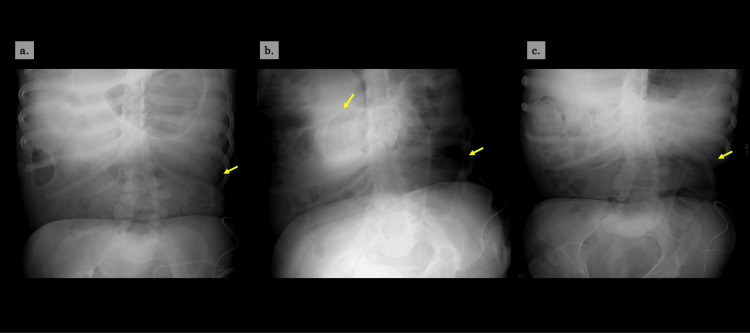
Changes in abdominal X-ray findings a. Postoperative day 7, no bowel dilatation b. Postoperative day 11 (before initiation of the stoma procedure), bowel dilatation extending c. Postoperative day 13 (after initiation of the stoma procedure), improvement trend in bowel dilatation
